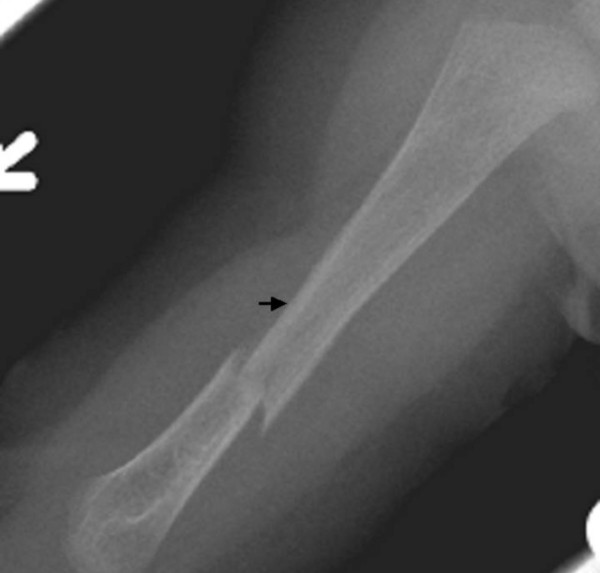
(patient 1). An anteroposterior radiograph of the right humerus showed mid-diaphyseal fracture and unusual diaphyseal endosteal hyperostosis (arrow).
